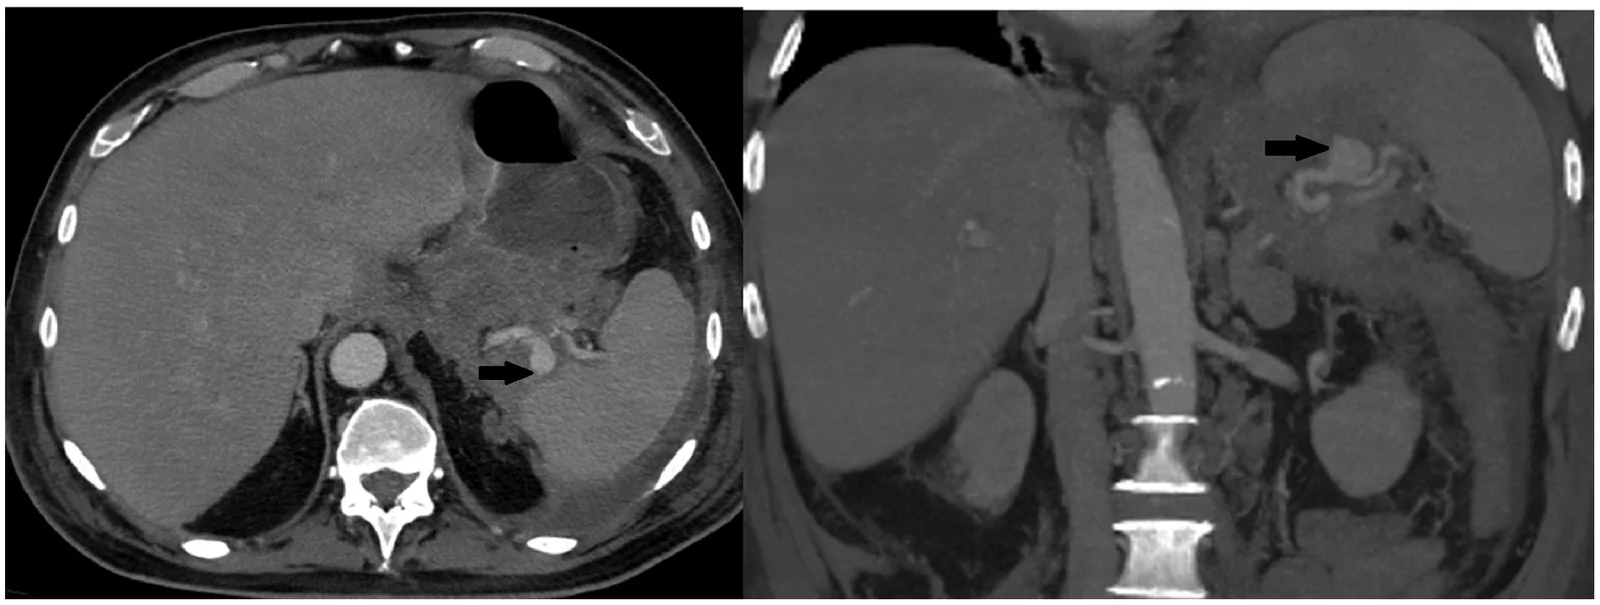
Arterial fase. CT with intravenous contrast performed on August 8, 2023 showing a 22-mm hyperenhancing nodular lesion apparently in continuity with the splenic artery (arrow). The location and arterial enhancement initially raised suspicion of a splenic artery pseudoaneurysm.
